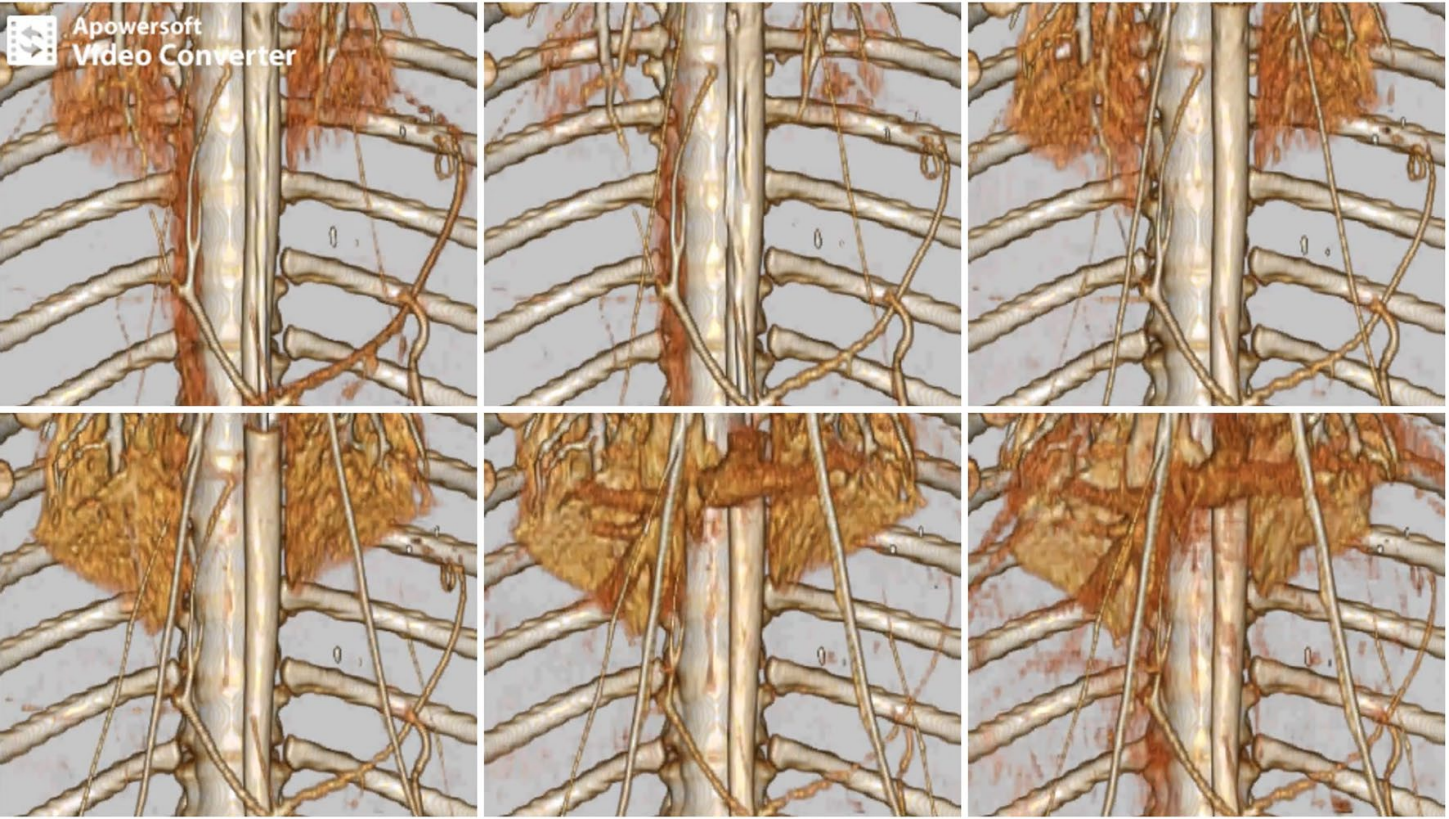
Volume-rendering images of dynamic four-dimensional computed tomography in haemorrhagic shock.
